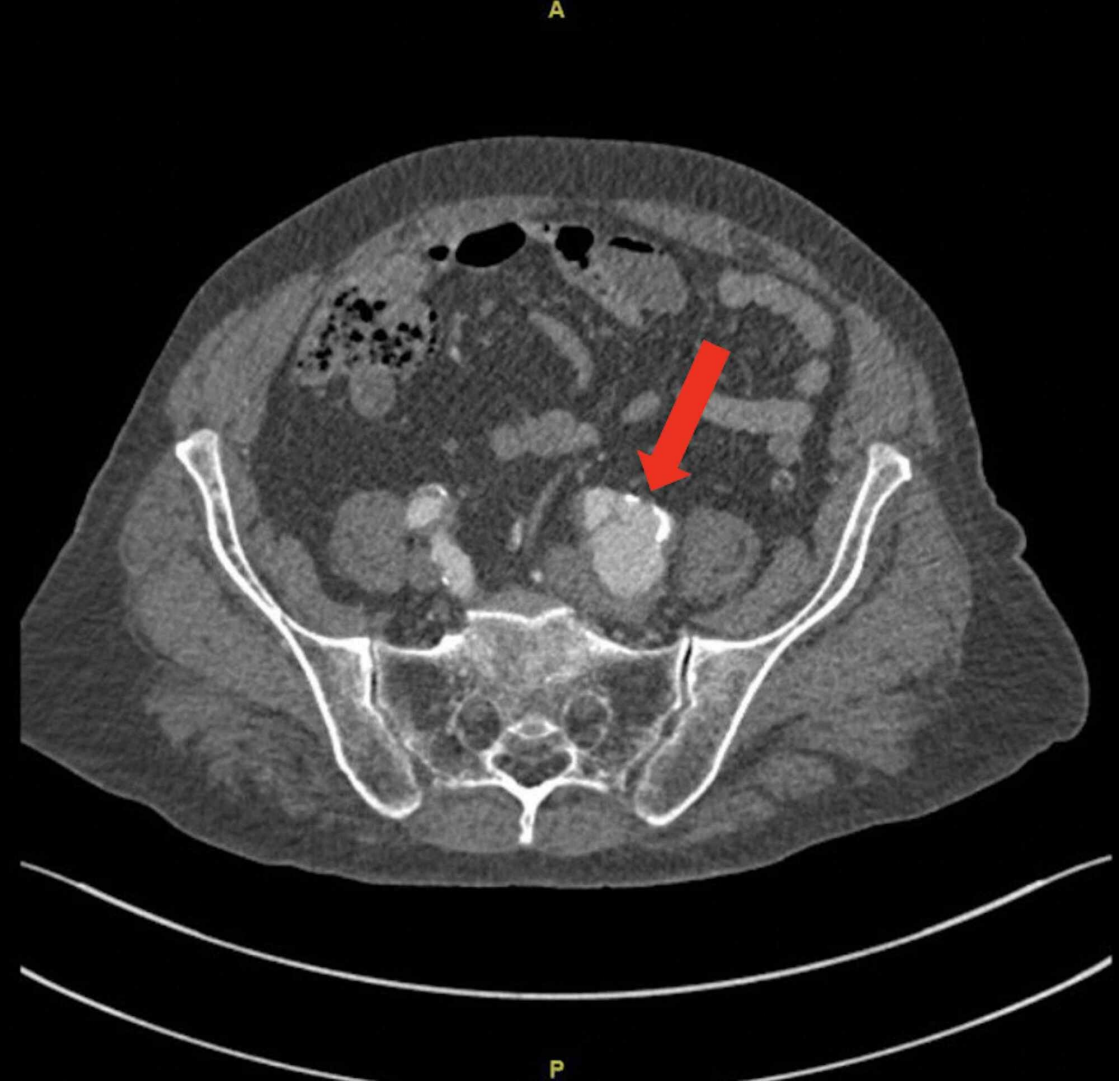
CT with contrast showing an isolated unruptured left common iliac artery aneurysm CT: computed tomography
